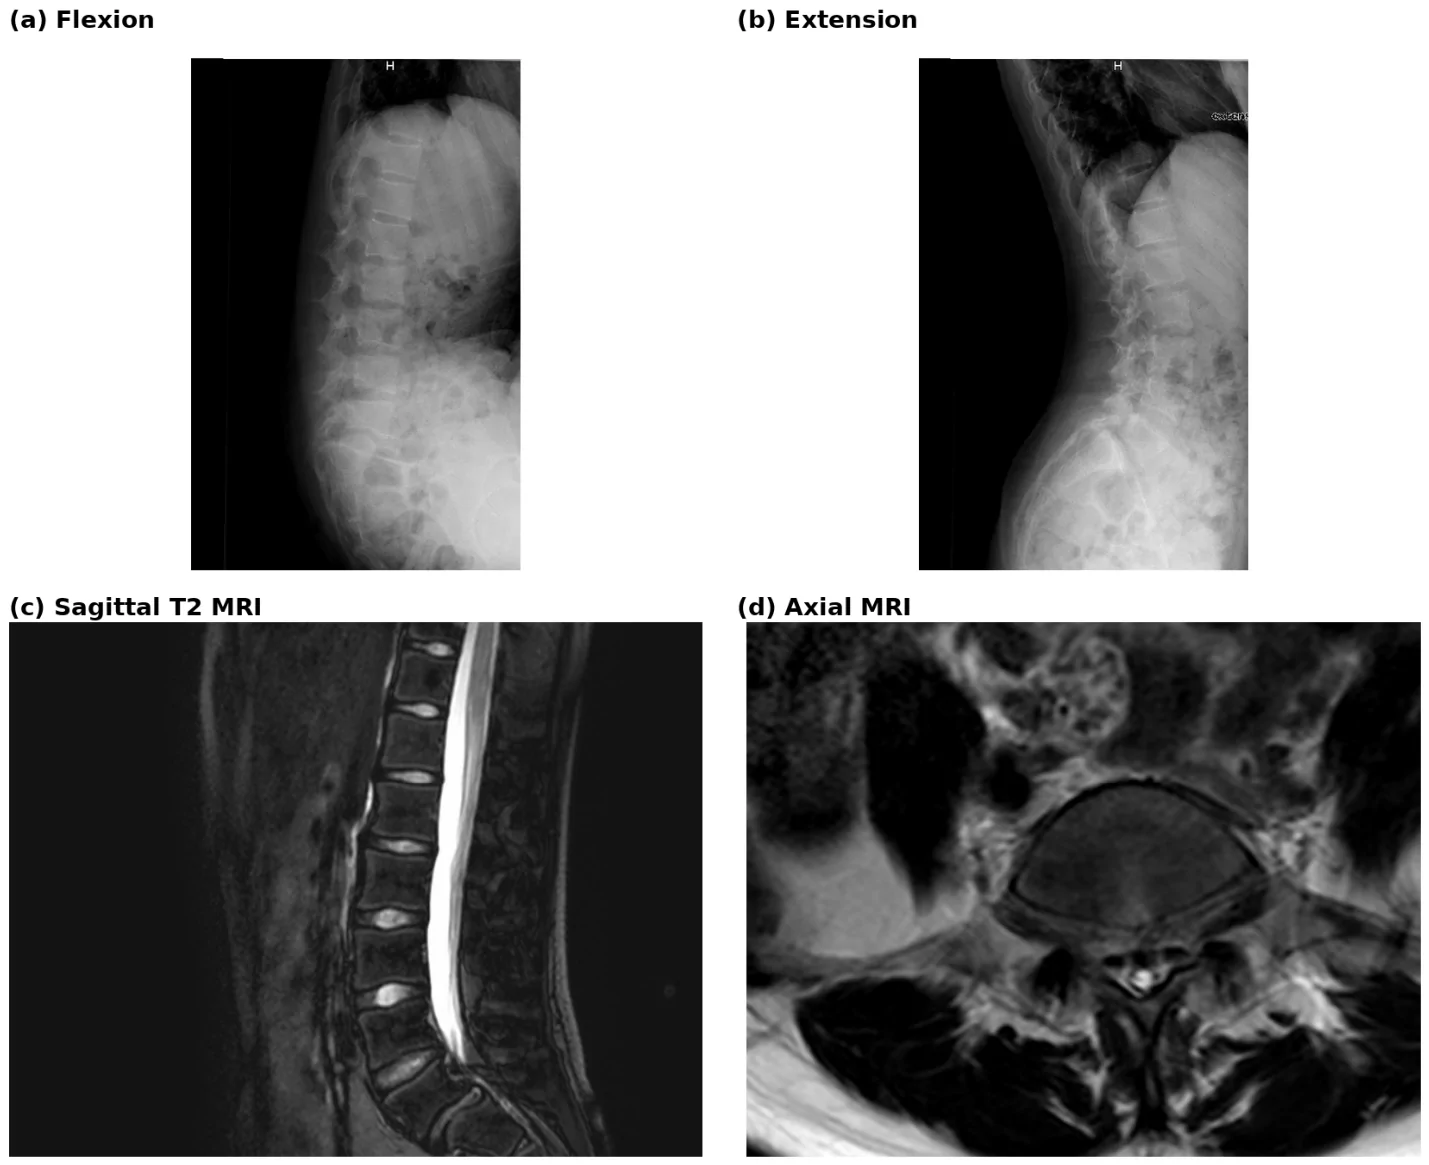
Flexion–extension radiographs and preoperative magnetic resonance imaging (MRI). (a,b) Flexion and extension lateral radiographs demonstrate measurable motion at L5-S1; the supplied segmental-angle measurements changed from −6.7° to +3.5° (10.2° excursion). This finding is reported as segmental mobility and is not interpreted as a validated threshold of pathologic instability. (c) Sagittal T2-weighted MRI and (d) axial MRI demonstrate the low-grade L5-S1 slip without a large focal disc extrusion, major central canal stenosis, or definite focal neural compression.
